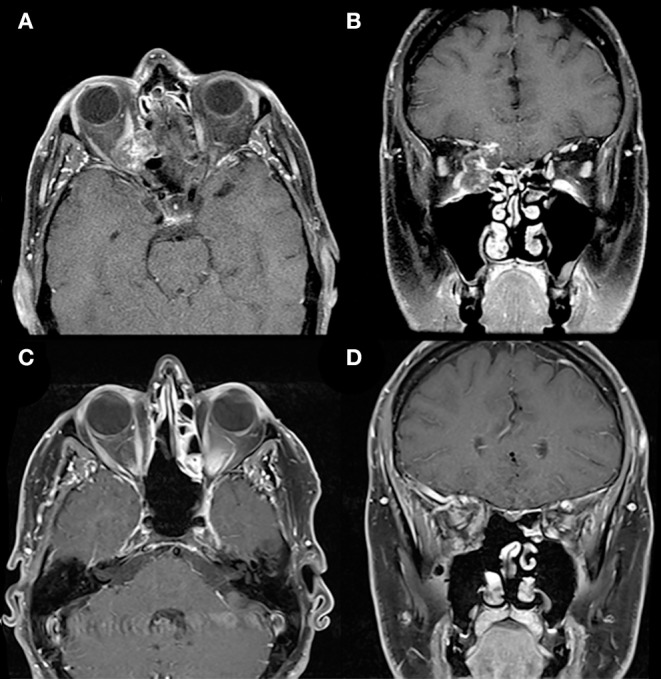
Extended endoscopic endonasal approach. Preoperative T1-weighted axial (A) and coronal (B) gadolinium-enhanced MRI demonstrate a right heterogeneously enhancing lesion involving the medial orbit, eroding through the lamina papyracea medially and the anterior skull base superiorly, but respecting the maxillary sinus inferiorly. The lesion compresses the right orbital contents including the optic nerve. The medial location of the lesion and erosion into the ethmoid sinus facilitated an endoscopic endonasal transmaxillary transorbital approach by a multidisciplinary team. Postoperative T1-weighted axial (C) and coronal (D) gadolinium-enhanced MRI demonstrate complete resection of this lesion.
